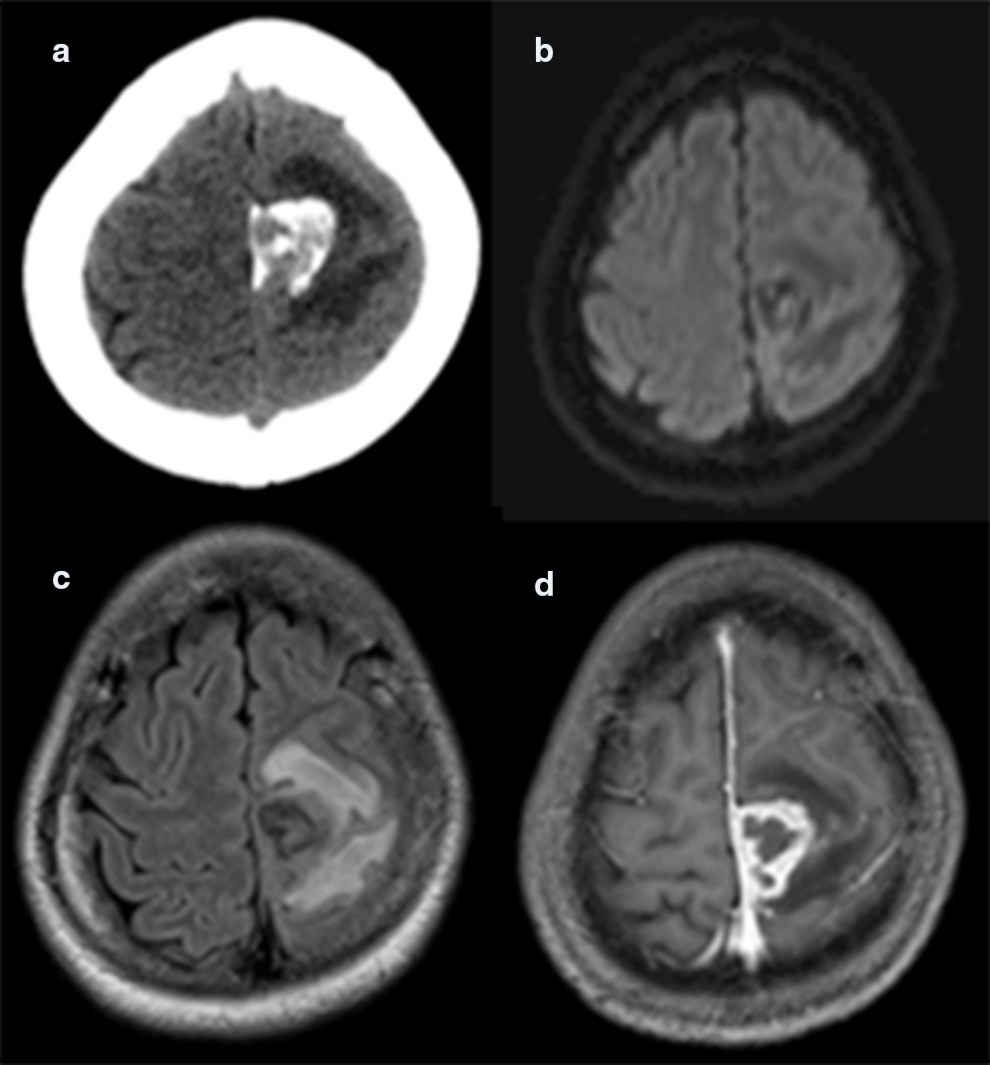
MRI and CT image of Case 2 demonstrating a left extra-axial parafalcine mass compressing the pre- and postcentral gyri and abutting the paracentral lobule. The CT image (a) shows heavy calcification and associated oedema. Peritumoral oedema is further demonstrated on the fluid attenuation inversion recovery image (c) with no diffusion restriction (b). The contrast-enhanced *T*1 weighted image (d) shows a marginally enhancing parafalcine mass with associated dural tail.
